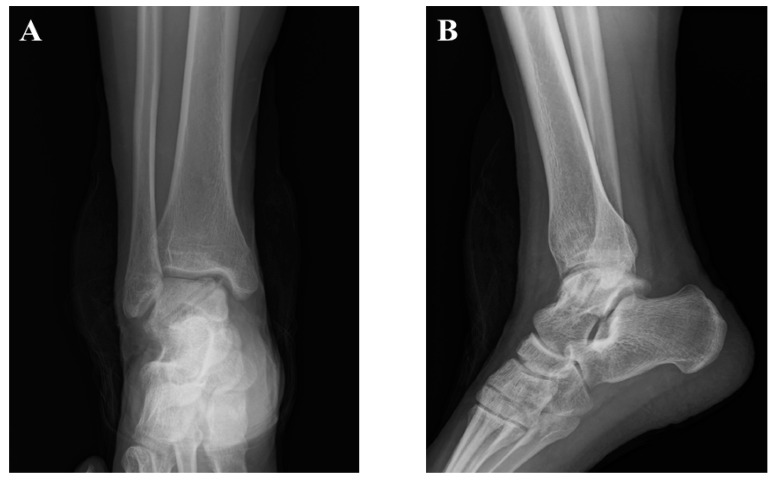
Post-operative radiographic images. (A) Antero-posterior view; (B) lateral view of the right ankle.
